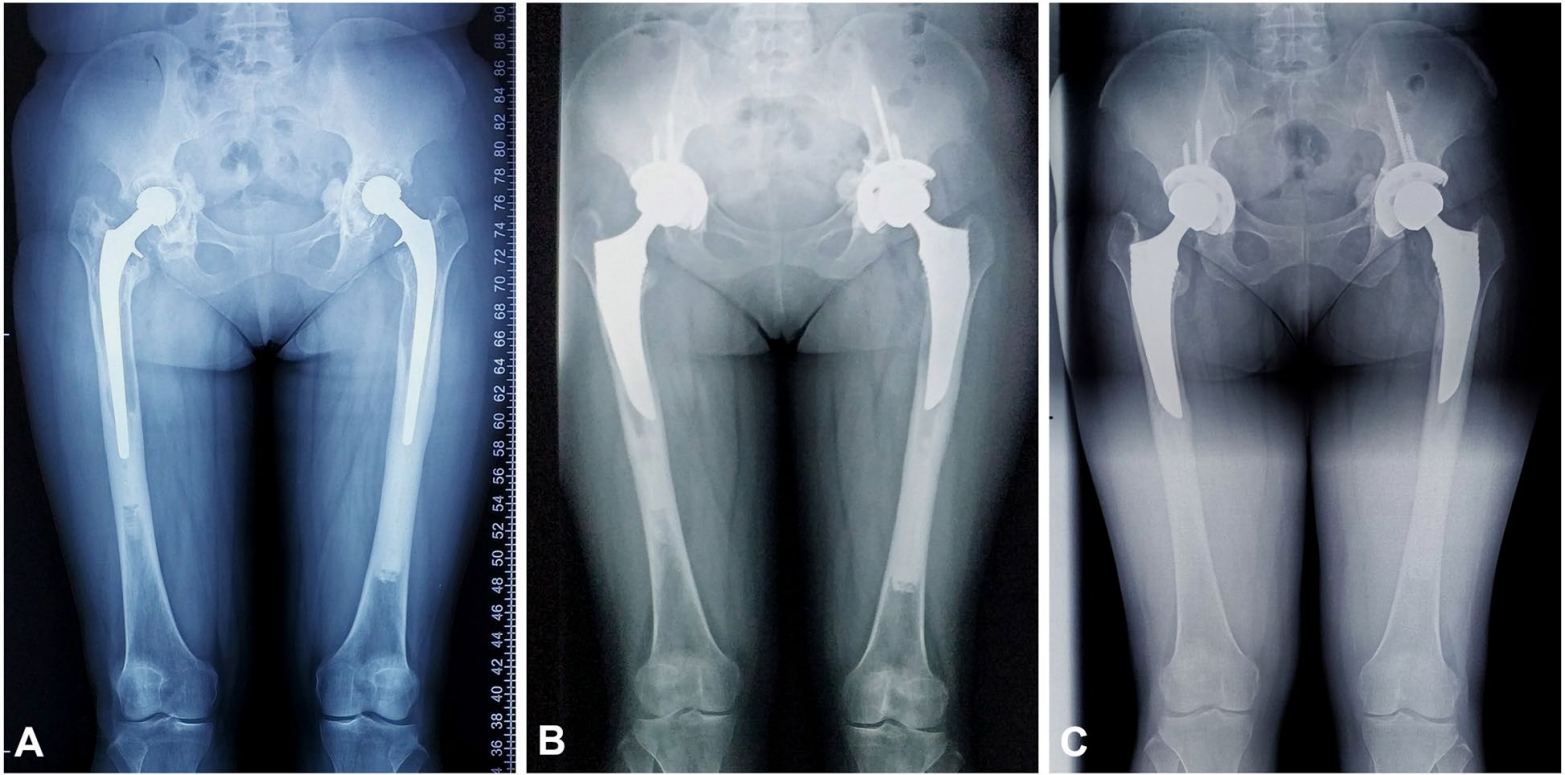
Radiographs show A proximal femoral bone defects (Paprosky IIIB in the right hip and IIIA in the left hip) in a 45-year-old female patient with aseptic loosening, B, C the fixed cementless alpha-conserve type I stem without radiolucent lines, osteolysis, or migration at 3- and 60-month follow-up
